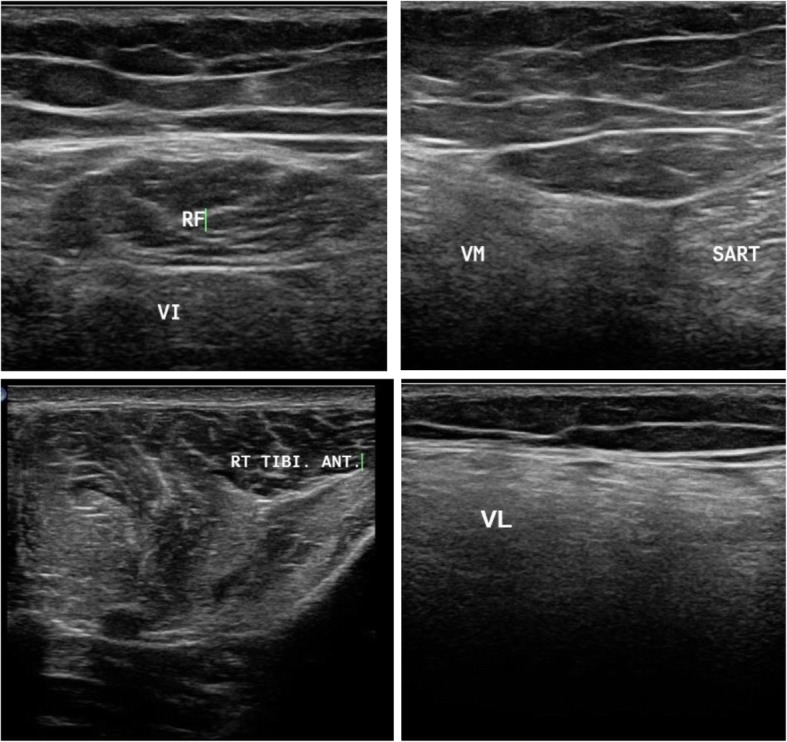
Muscle ultrasound in patient suspected to be with acid maltase deficiency showing affection of the vastus intermedius (VI) and sparing of the rectus femoris (RF) and vastus lateralis (VL) and affection of the vastus medialis (VM) with sparing tibialis anterior (TA)
